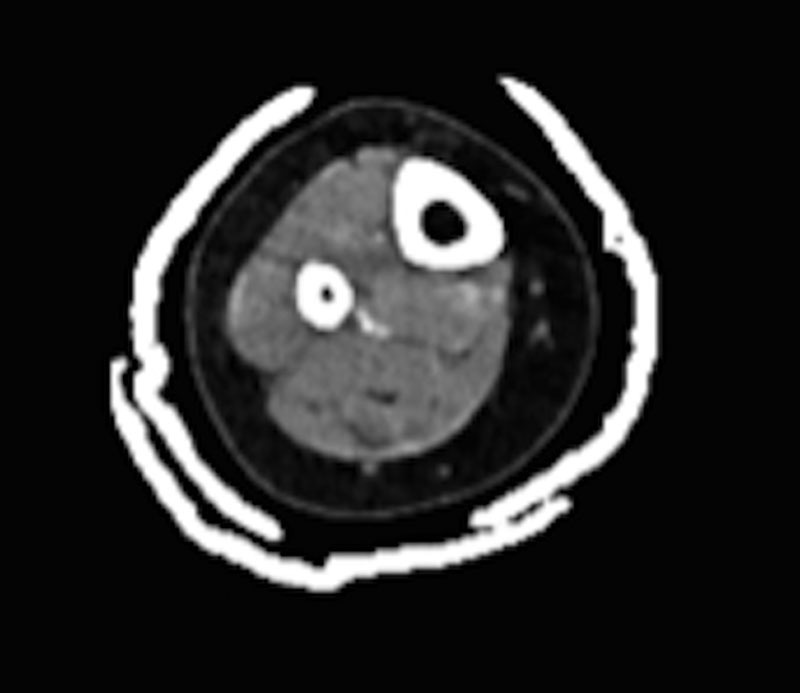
CTA demonstrating laterally based peroneal artery perforator supplying medial sided skin. Perforator coursing medially between deep and superficial posterior compartments.
